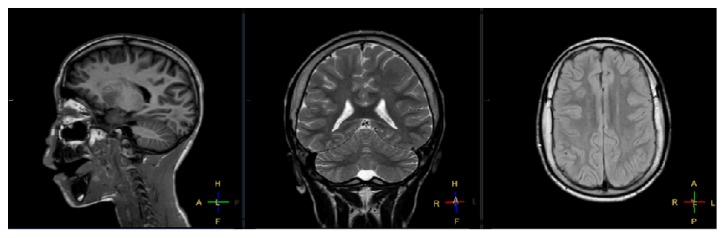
Brain MRI (sagittal, coronal, and axial views) showed a diffuse hyperintensity of supratentorial white matter, a moderate enlargement of the lateral ventricles, a moderate expansion of hemispheric grooves, and a thickening of the cranial vault.
